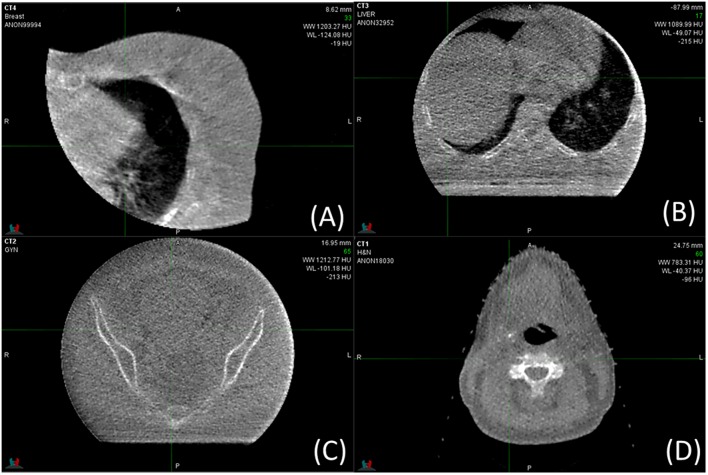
MV-CBCT images obtained for clinical treatments of different sites. Display parameters are: (A) WW: 1,203, WL: −124, (B) WW: 1,090, WL: −49, (C) WW: 1213, WL: −101, (D) WW: 783, WL: −40, (WW, window width; WL, window level).
